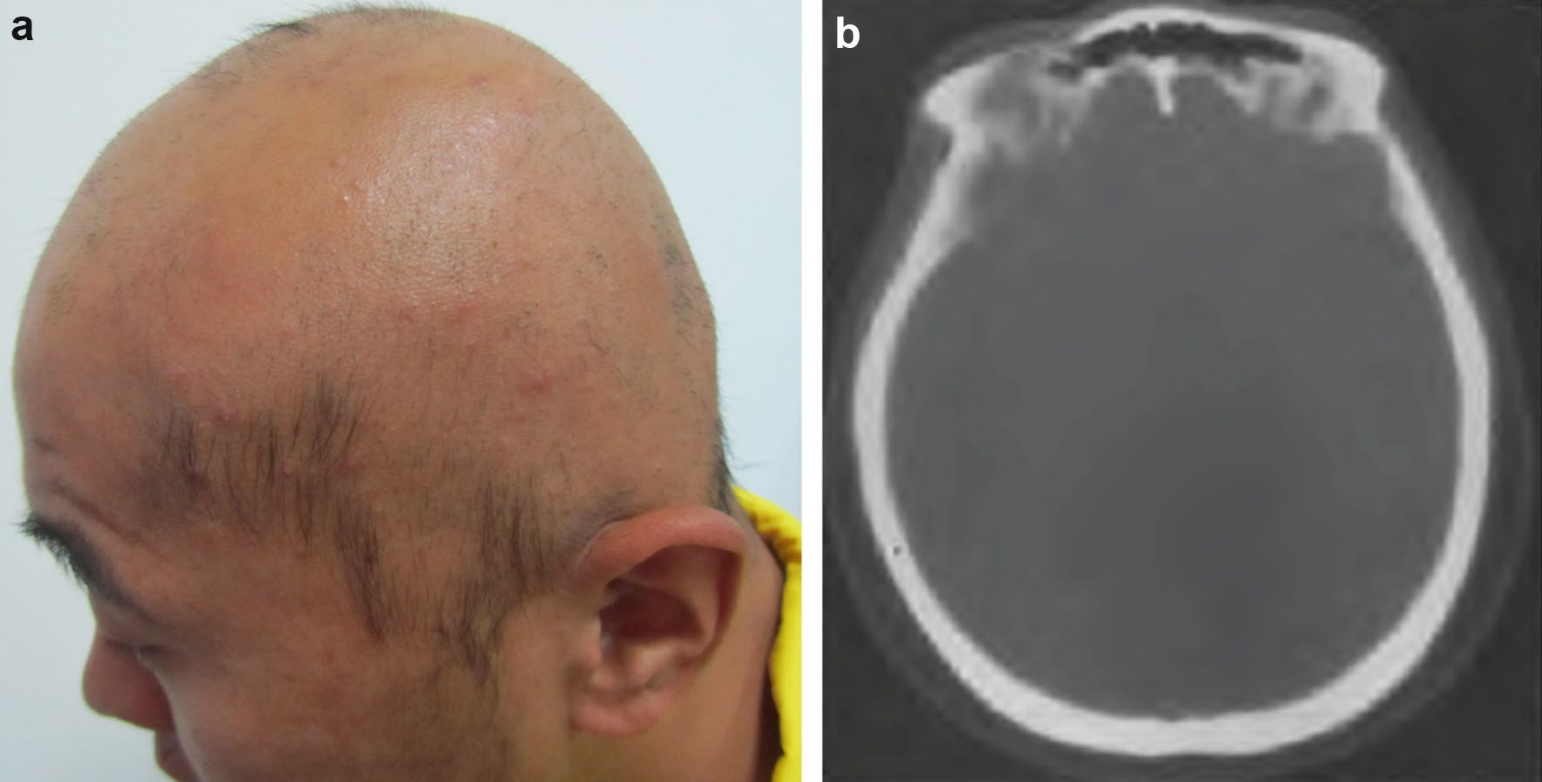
a Clinical manifestation showing alopecia accompanied by thickened scalp. b CT scan showing thickening scalp with marked expansion of subcutaneous adipose tissue approximately 15 mm in the occipital region
